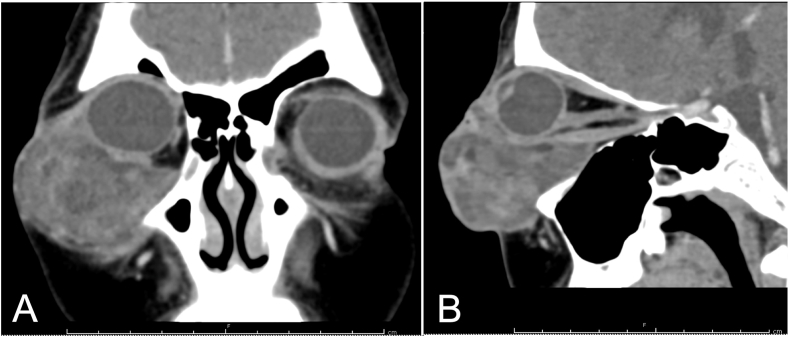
Orbital CT scan of the patient after the 4th cycle of chemotherapy. (A) Coronal view without contrast. (B) Sagittal view without contrast.
